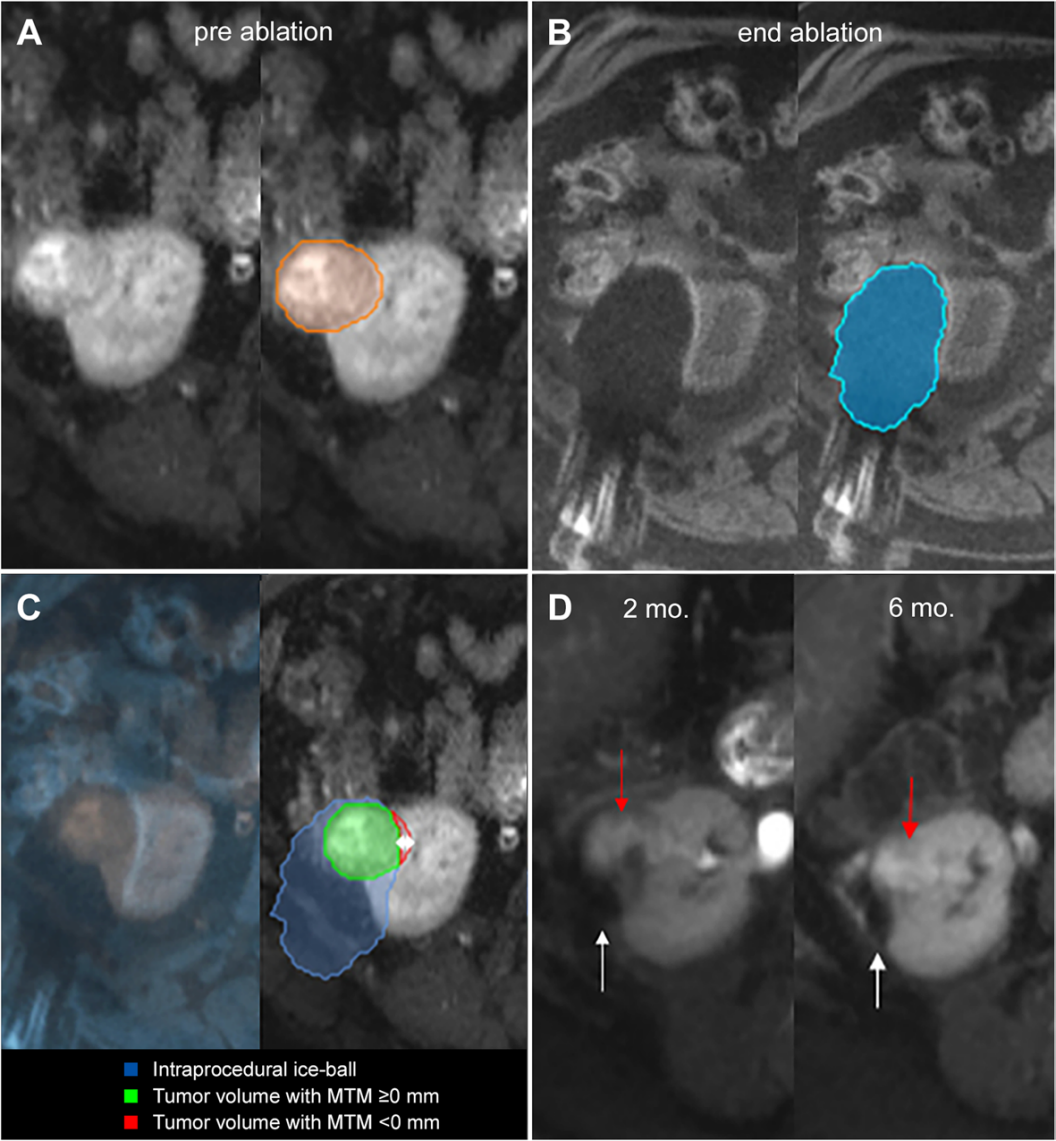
A Segmentation of a 4.2 cm diameter tumor located in the lower pole of the right kidney (outlined in orange) on pre-ablation axial T2-weighted MR imaging. B Segmentation of the corresponding ice ball (outlined in blue) on post-ablation axial T1-weighted MR imaging. C Overlay of pre-ablation and post-ablation images after co-registration (left) and resulting 3D margin calculation (right). Volumetric assessment shows predicted incomplete ablation at medial side (red) with MTM of − 6 mm. D Follow-up axial T2-weighted MR imaging at 2 months (left) after cryoablation shows ablation zone (white arrows) with suspected incomplete coverage on medial side (red arrows). Six months follow-up MRI (right) confirms local tumor progression (red arrow) at the medial side
